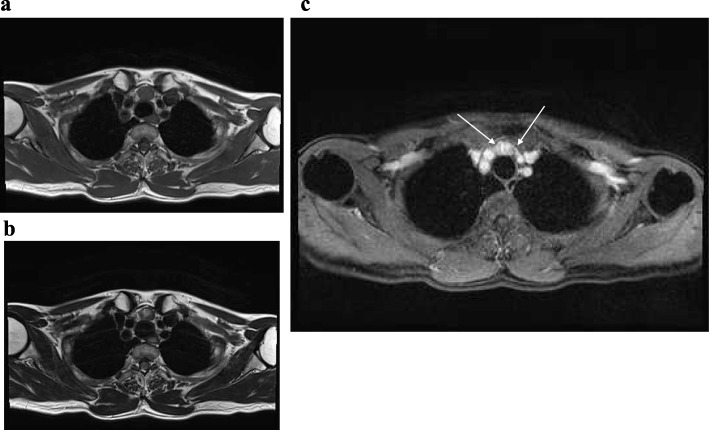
MRI images of ectopic mediastinal thyroid tissue. a Low signal intensity on T1-weighted images. b High signal intensity on T2-weighted images. c Enhanced MRI showing stronger enhancement. Arrows show tumor-feeding arteries arising from the vessels in the thorax
